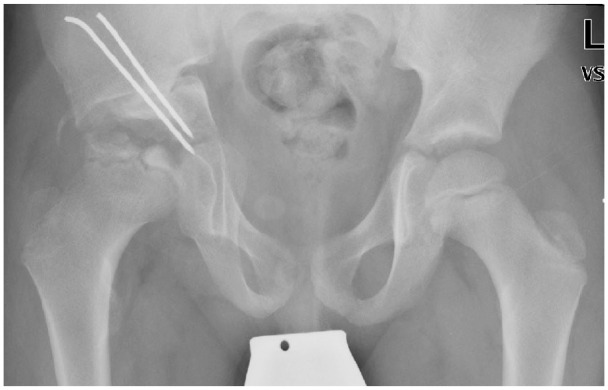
Postoperative radiograph of a 10-year-old boy after Chiari pelvic osteotomy for severe LCPD of the right hip.
